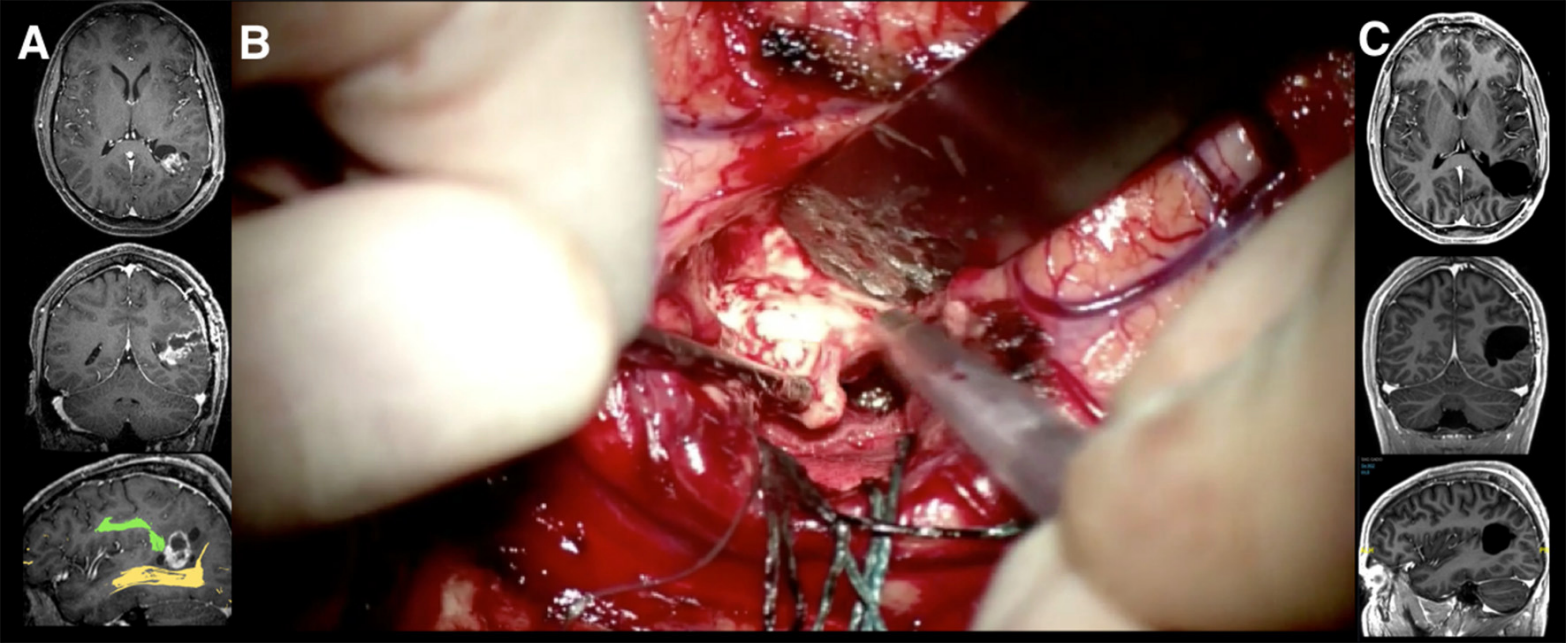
A 33-year-old man who presented with a headache was treated by awake surgery for a Pleomorphic Xanthoastrocytoma after an open biopsy was performed in a referral center. (A) The T1 weighted MR images after gadolinium injection in axial, coronal, and sagittal planes are shown. The lesion is located in the left temporoparietal area, just dorsal to the telencephalic flexure. This area shows highly eloquent cortical regions around the terminal part of the Sylvian fissure. The tumor grows from the cortical surface of the angular gyrus toward the ependymal wall of the left atrium. This area presents an underlying complex network of white matter tracts, the intimate relationships of which must be studied preoperatively. Some of these fibers show a curved shape as a result of the telencephalic flexure appearing due to the physical and genetic forces that made our brains more functionally advanced. Thus, the sagittal image shows its close relationship with the fused DTI-tractography images of the arcuate fascicle *(green)* and the optic radiations *(yellow*), which seemed to be displaced by the tumor itself. The neurological examination was completely normal including the visual field campimetry (B). Due to the age of the patient and tumor location, an awake craniotomy was performed with the aim of testing the language and visual function during the tumor resection. The speech was tested to increase the tumor resection anteriorly and no deficit was noted even when reaching the most anterior part. During resection of the deeper parts of the tumor, intra- operative visual tests were performed. Stimulations to different points along the optic radiations evoked reversible scotomas in different portions of the visual field as described by the patient. At the completion of the optic radiation mapping, the resection of the tumor was performed radically in those areas where the vision remained normal despite subcortical stimulation. (C) The postoperative MRI at three months revealed a complete resection of the tumor. Postoperative campimetry at three months revealed a minor homonymous deficit in the right inferior quadrant.
